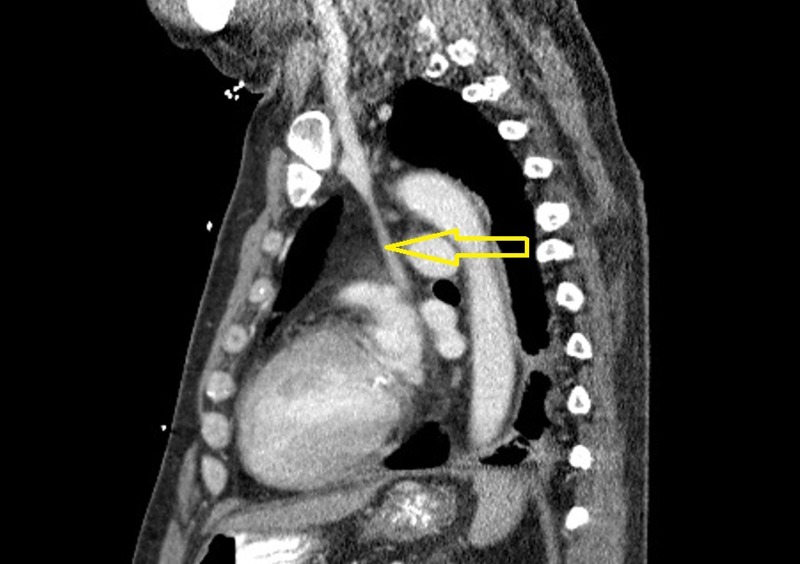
Chest computerized tomography (CT) scan (sagittal view) with contrast performed on a prior admission confirming the presence of a duplicated superior vena cava (SVC) (marked by yellow arrow) draining into the coronary sinus.
